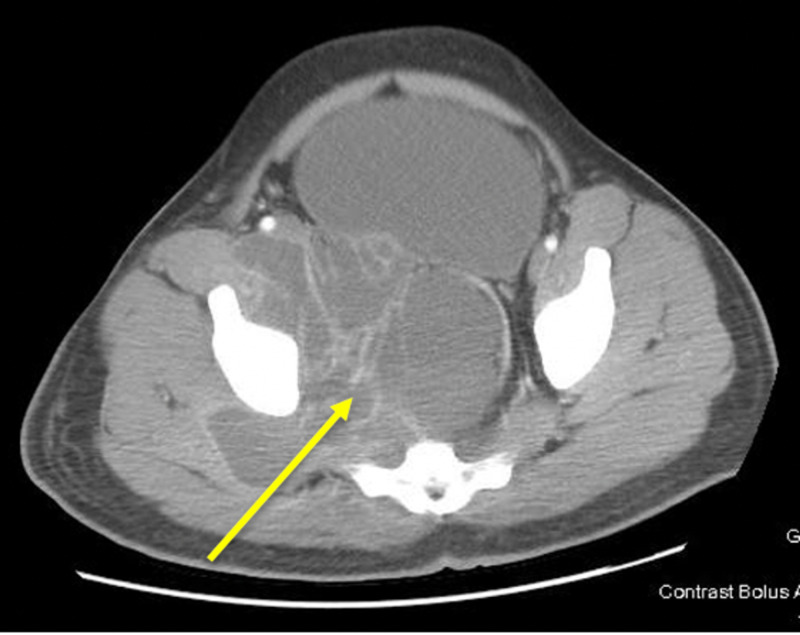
Transverse CT section demonstrating a 14 x 17 cm cystic mass with extra-pelvic extension (yellow arrow).
